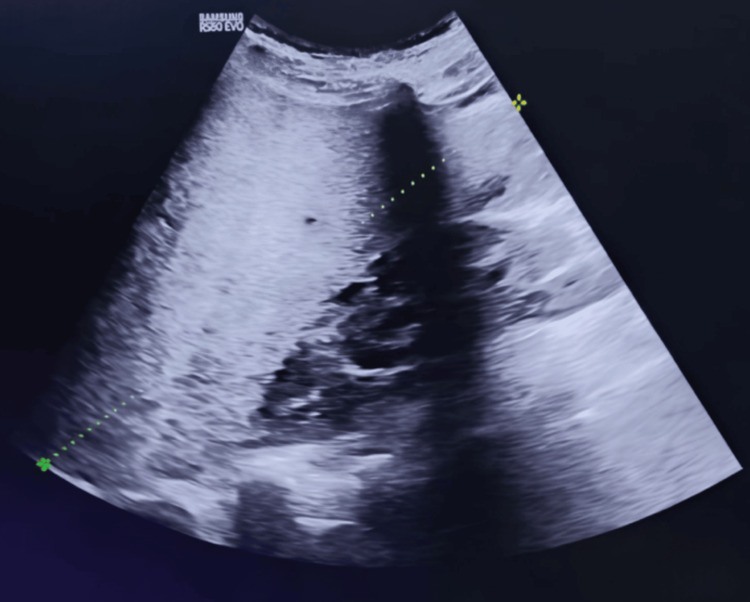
Ultrasound Showing Gross Ascites Abdominal ultrasound revealing anechoic fluid collection consistent with gross ascites, supporting the diagnosis of Budd-Chiari Syndrome in a patient with paroxysmal nocturnal hemoglobinuria.
